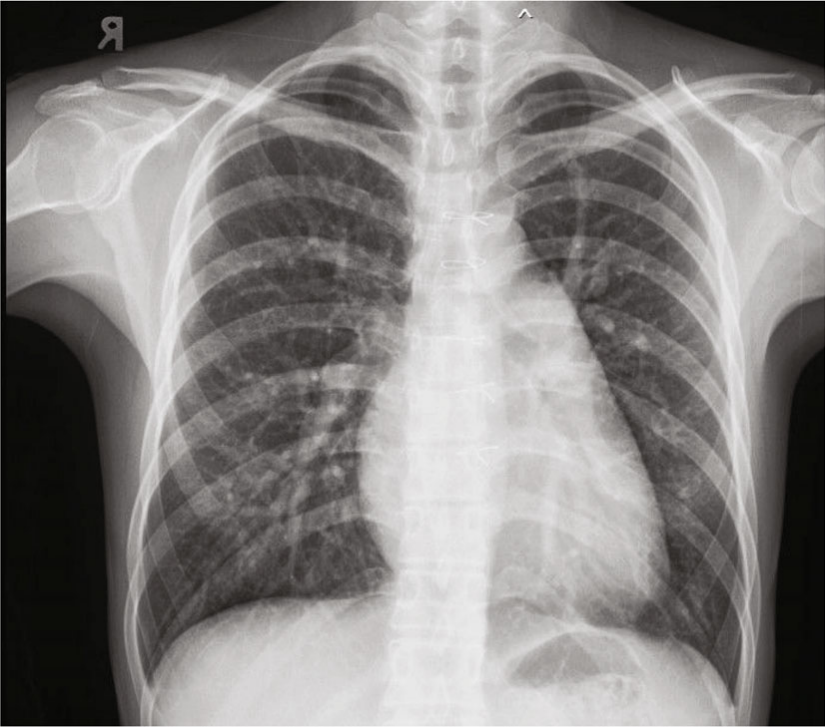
Plain radiograph of the chest showing mild cardiomegaly with left atrialization and slight increase in pulmonary blood flow.
